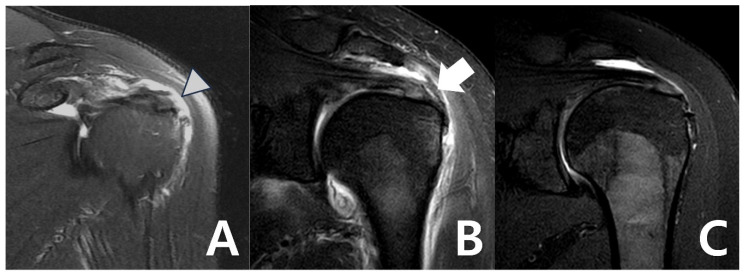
(A) Bursal-side partial-thickness supraspinatus tear with subacromial spur was observed on the preoperative magnetic resonance imaging. (B) Bioinductive collagen implant located on the tendon was observed in a postoperative magnetic resonance image. (C) A magnetic resonance image 6 months after surgery showed that the bio-inductive collagen implant was absorbed, and the bursal-side partial tear was fully recovered.
